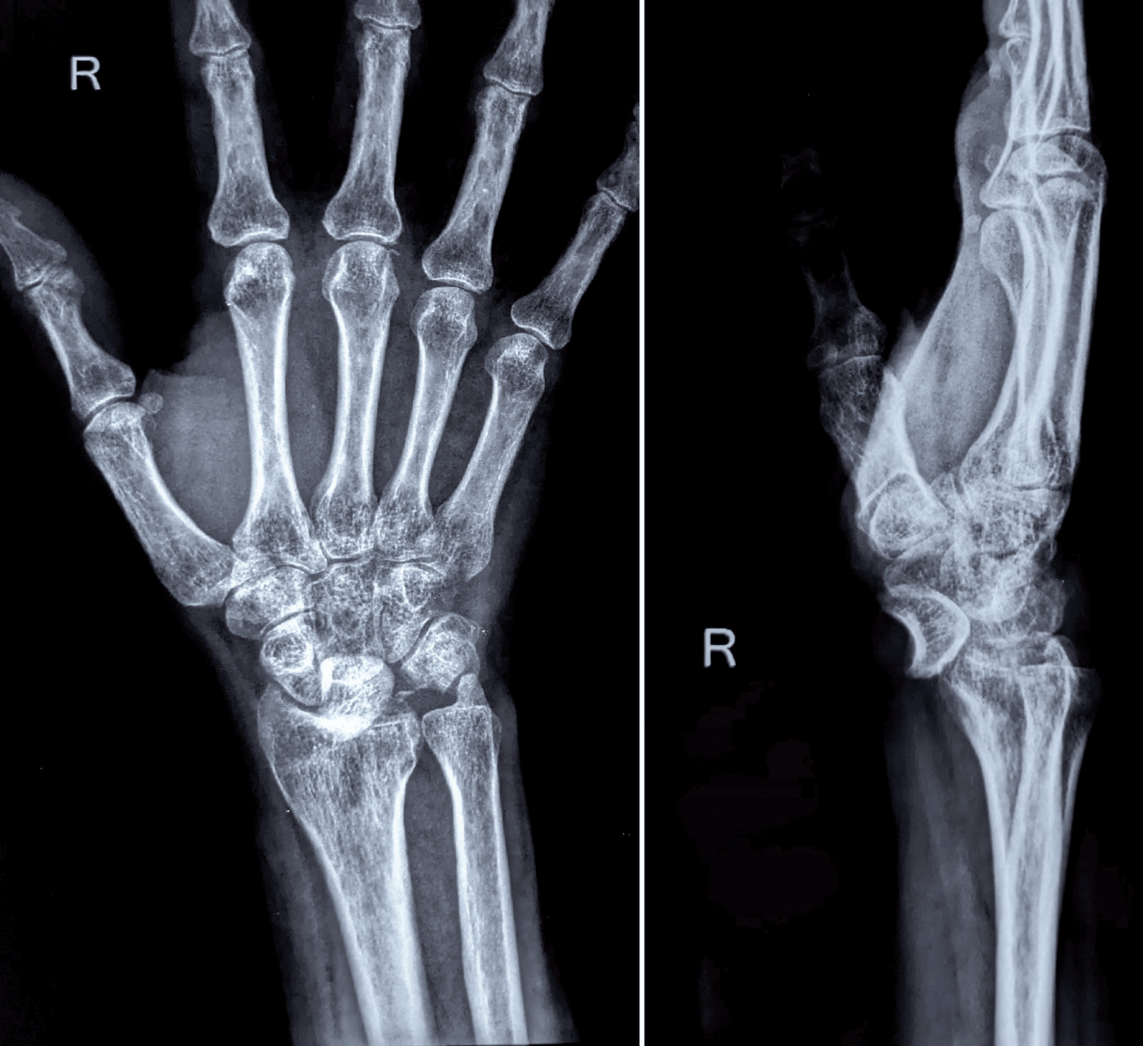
Radiographs of the right wrist showing volar dislocation of the lunate (spilled tea-cup sign) with an avulsion fracture of the triquetrum.
